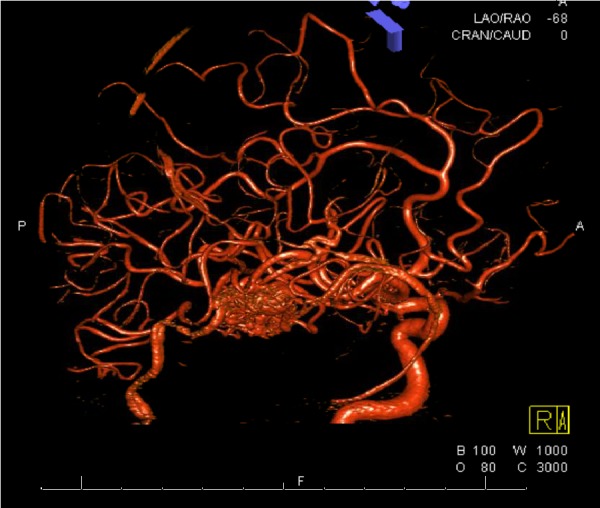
3D Computed Tomography Angiogram Reconstruction 3D - three dimensional
